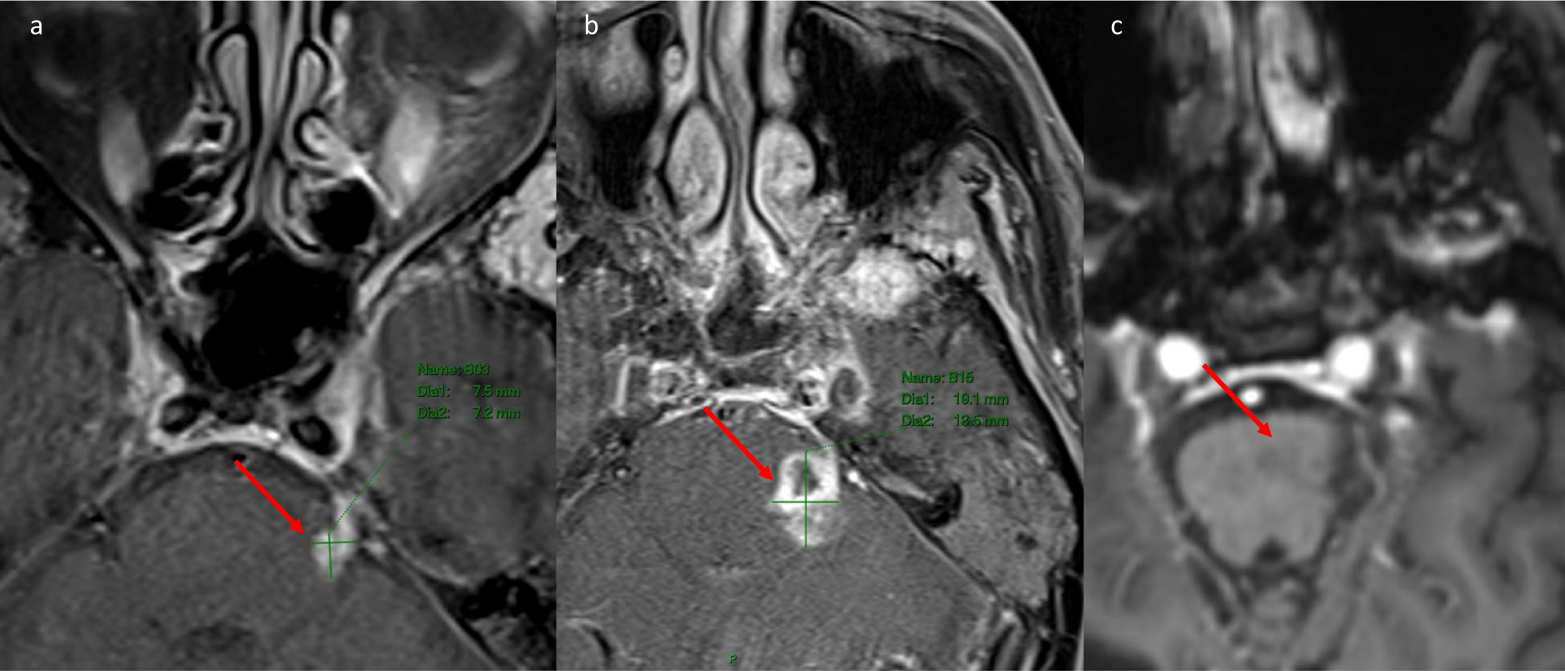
Pseudoprogression demonstrated on magnetic resonance imaging (MRI). MRI axial T1 post-contrast fat saturation (FS) axial demonstrates: (A) baseline perineural spread from cutaneous squamous cell carcinoma (CSCC) with mass enhancement at left pons and cisternal portion of the left trigeminal nerve. B < 5 months, on immunotherapy, enlarged mass-like change in the left trigeminal nerve with increased enhancement at the left pons and left Meckel’s cave. C > 10 months, (26 months) post immunotherapy, the left pons mass has resolved and the thickening and enhancement of the left trigeminal nerve has resolved
